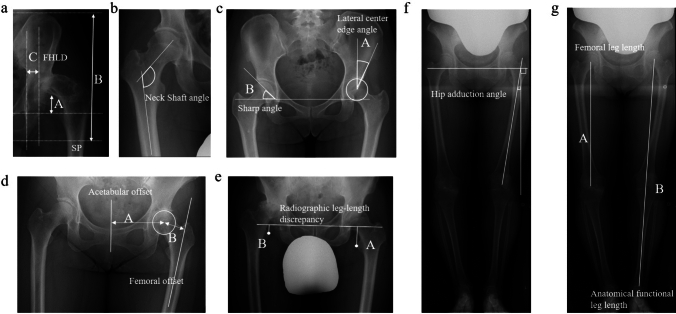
Radiographic evaluation of the lower limbs. a Subluxation percentage: 5A/B, Femoral head lateralization distance: C. SP: Subluxation percentage, FHLD: Femoral head lateralization distance. b Neck shaft angle. c Lateral center edge angle: A, sharp angle: B. d Femoral offset: A, acetabular offset: B. e Radiographic leg-length discrepancy: A-B. f Hip adduction angle. g Femoral leg length: A, Anatomical functional leg length: B
